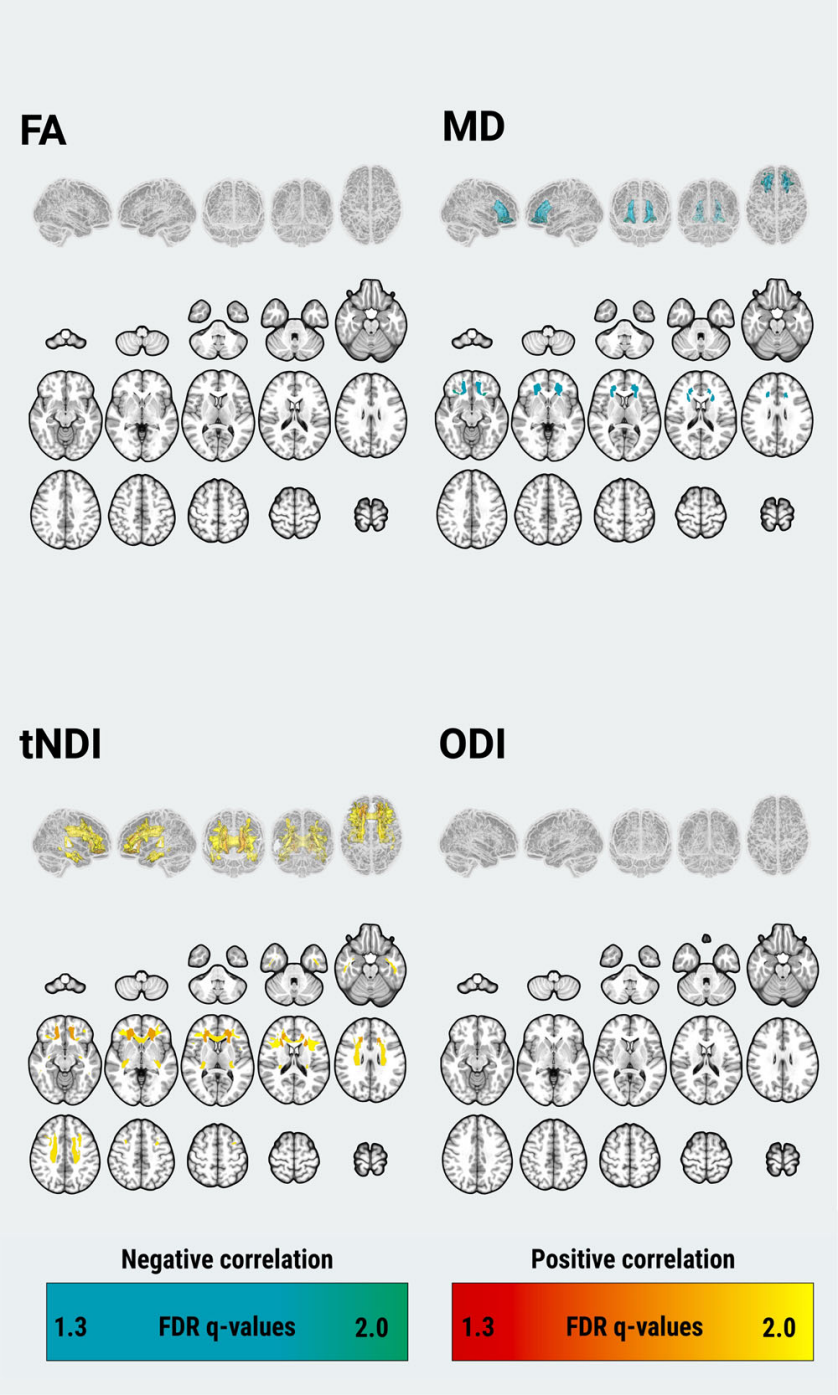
Relationship between tau and white matter microstructure in CU. Statistically significant associations of cortical [11 C]-PiB SUVr with regional DTI and NODDI parameters are depicted on 3D glass brain renderings and in volumetric MNI152 space (radiological orientation) for the CU controls. Linear regression models were adjusted for age, APOE genotype and [18 F]-Flortaucipir, and corrected for multiple comparisons with FDR. FDR q values are expressed as -log10(q) (i.e., 1.3 -> q = 0.05). Abbreviations: CU Cognitively unimpaired, DTI Diffusion tensor imaging, APOE Apolipoprotein E, FDR False Discovery Rate, FA Fractional anisotropy, MD Mean diffusivity, NODDI Neurite Orientation Dispersion and Density Imaging, tNDI tissue-weighted Neurite Density Index, ODI Orientation Dispersion Index, MNI152 Montreal Neurological Institute, SUVr Standardized uptake value ratios.
